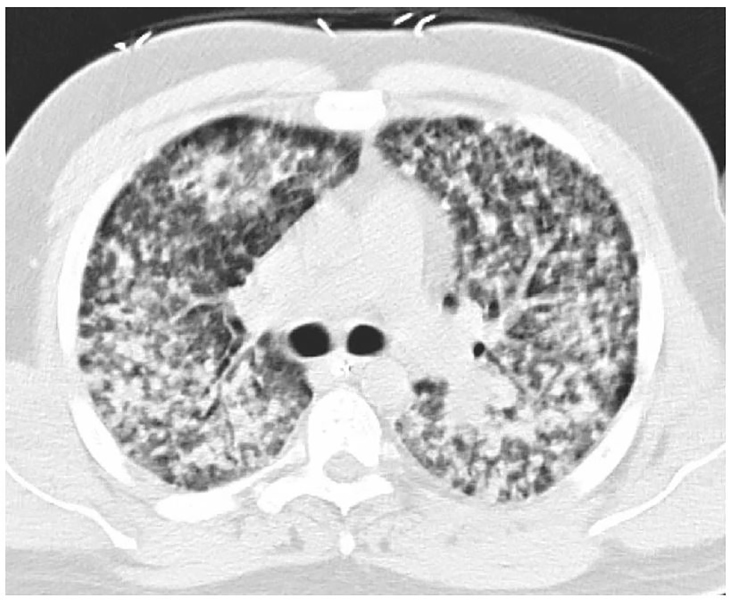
Axial image at the level of the mainstem bronchi taken from a computed tomography of the chest obtained in the emergency department, showing diffuse, nodular opacities in both lungs.
